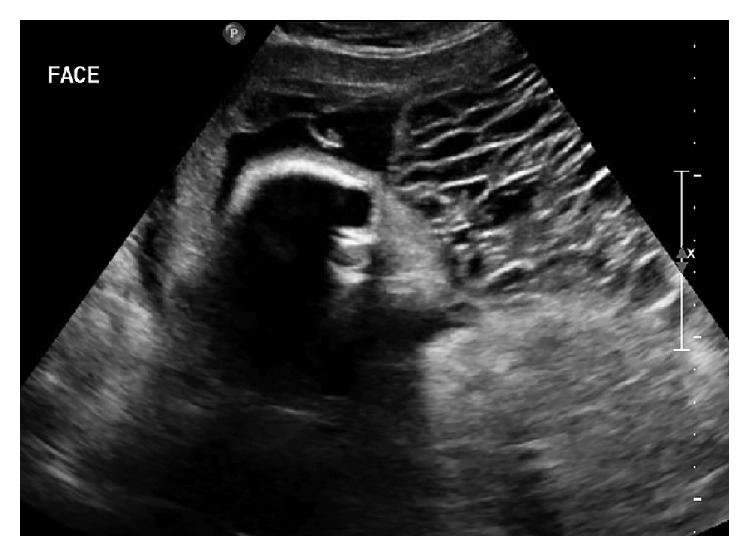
Ultrasound appearance of the fetus next to the molar component of the pregnancy at 26 weeks.
